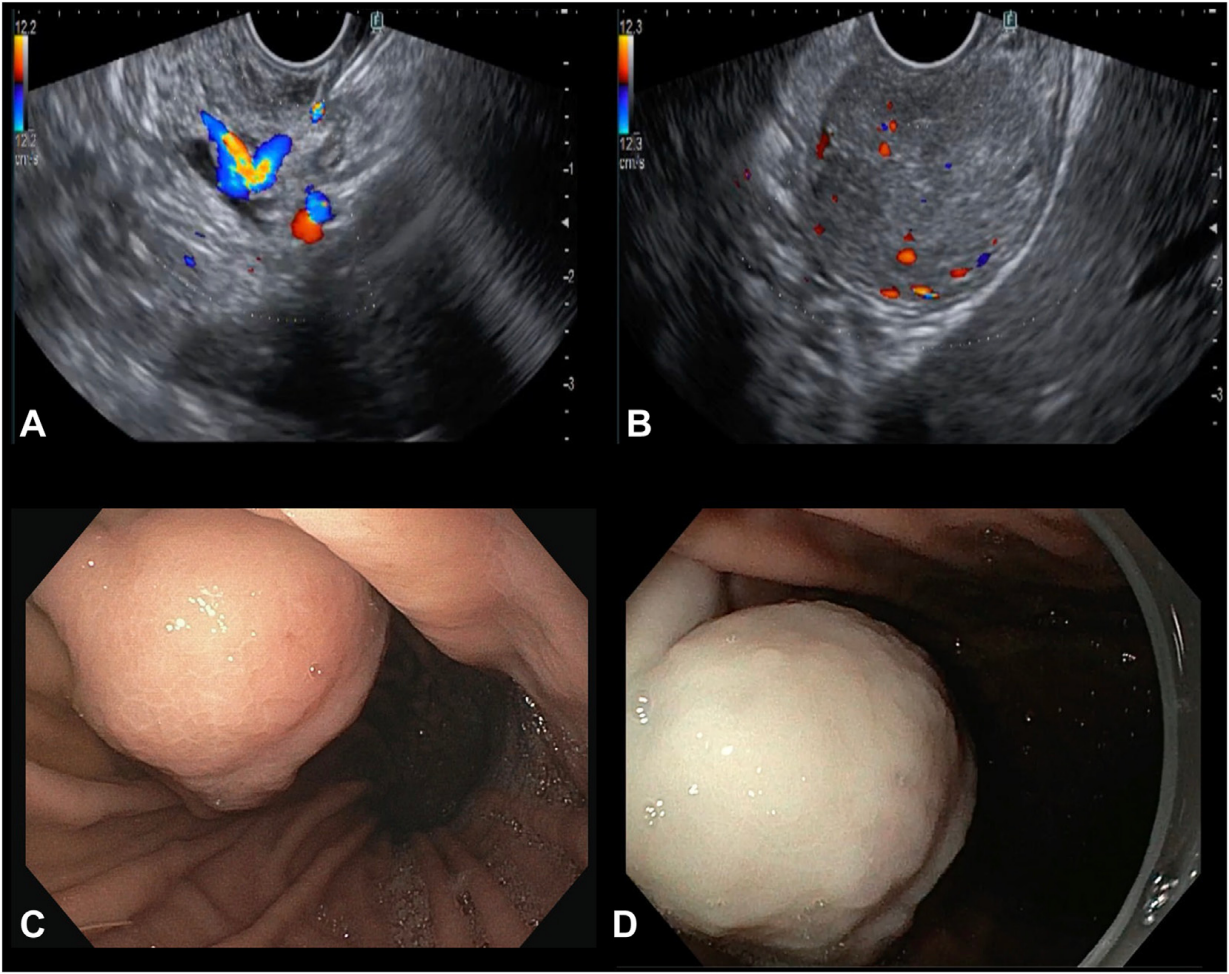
Pre-emptive EUS-guided epinephrine injection performed at the base into the feeder vessel. A, The feeder vessel was identified on EUS, and adjacent area was targeted with a 19-gauge needle, and diluted epinephrine injection (1:10,000) was administered. B, After epinephrine injection, a significant decrease in Doppler flow was noted. C, Gastroscopic view of the gastric lesion before epinephrine injection. D, Gastroscopic view of the gastric lesion postepinephrine injection, showing the lesion becoming pale.
